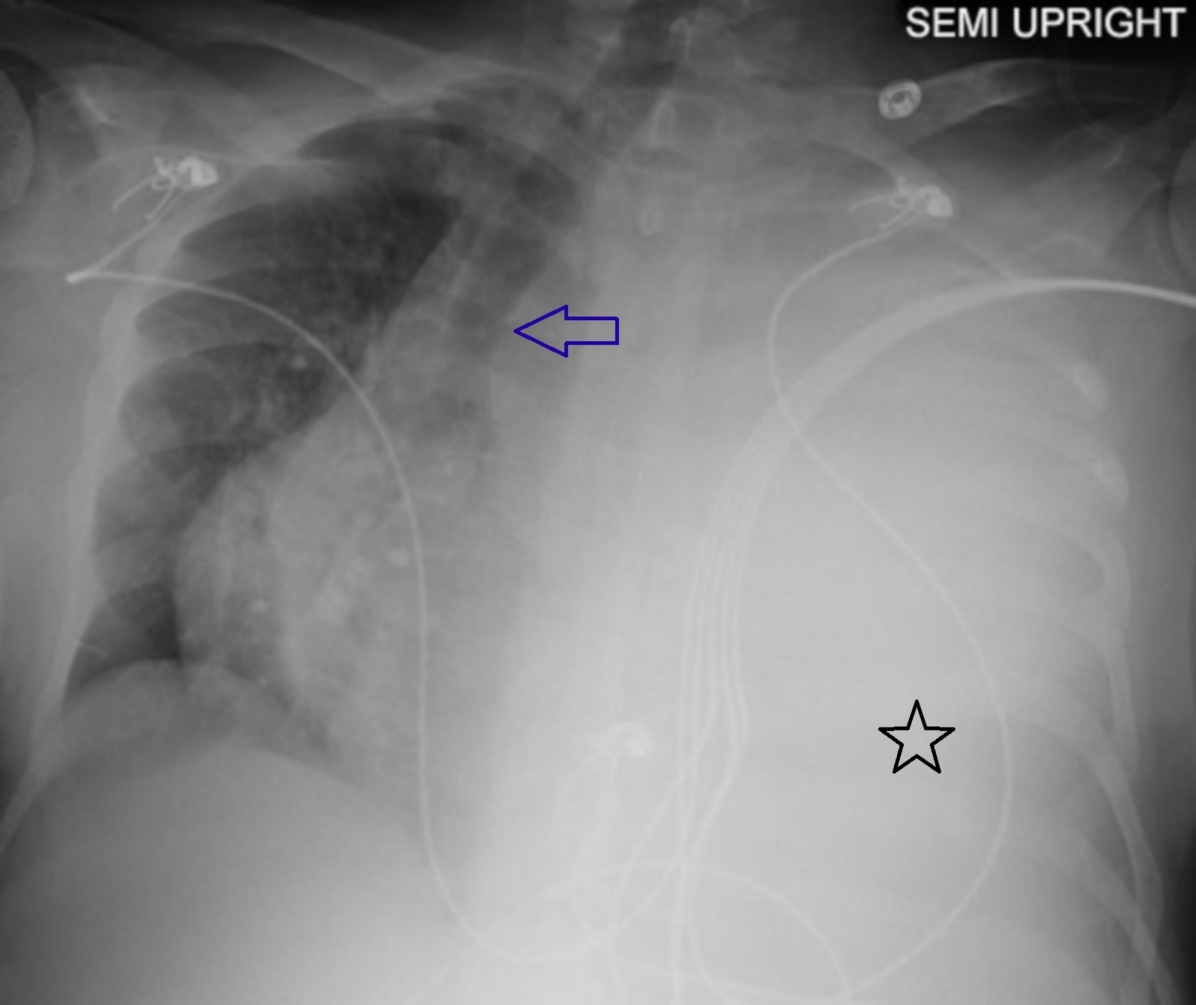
Left pleural effusion on chest X-ray Portable plain radiograph of the chest showing a massive left pleural effusion (black star) with contralateral displacement of trachea (blue arrow)
